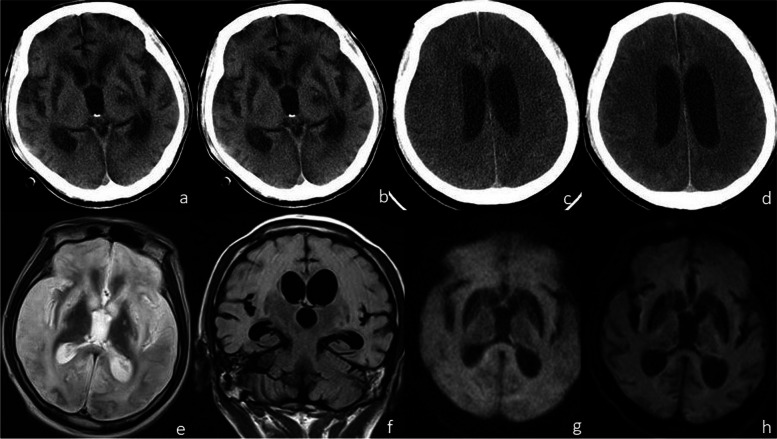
A 41-year-old male septic tank worker who fainted immediately and lost consciousness after H_2_S inhalation was rescued after 50 min but showed lip cyanosis, lock-jaw, and limb tics. He was still in a coma after symptomatic treatment in another hospital. He had a fever after 2 days. On admission, he was in a deep coma, the muscle strength of the four limbs was grade I, and the muscle tension of both arms was enhanced compared with normal. Plain CT scan of the brain revealed symmetrical stripes and patch hypodensities in the bilateral basal ganglia and around the lateral ventricles 45 days after H_2_S inhalation (a); CT of the brain was performed 60 days and 4 months after H_2_S inhalation (b and c), and generalized brain edema appeared and continued to aggravate (CT values were 25 and 17 HU, respectively); CT was performed again after 5.5 months (d), on which the severity of brain edema was slightly alleviated, and partial corticomedullary differentiation was slightly clearer than before; on MRI of the brain 4 months after H_2_S inhalation (e-g), the bilateral basal ganglia were symmetrically hypointense on T1WI and hyperintense on T2WI (e), the brain edema showed diffuse hyperintense signals on T2WI and involved the cortex and the white matter, T2WI-Flair (f) and DWI (g) revealed diffuse hyperintensities, and the basal ganglia lesions were hypointense; MRI of the brain performed after 5.5 months (h) revealed a slightly lower degree of hyperintensity on DWI than before. The patient was persistently in an agrypnocoma during follow-up
